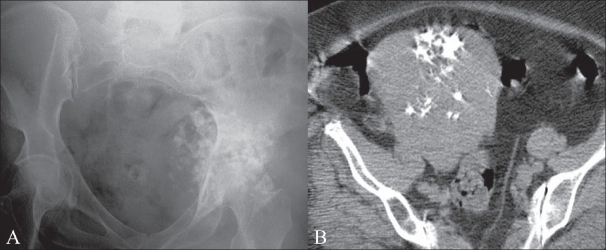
A 58-year-old woman with sacral pain. A frontal pelvic radiograph (A) shows calcification overlying the left hip, initially thought to be suggestive of a chondrosarcoma. A subsequent CT scan (B) reveals an incidental calcified fibroid
